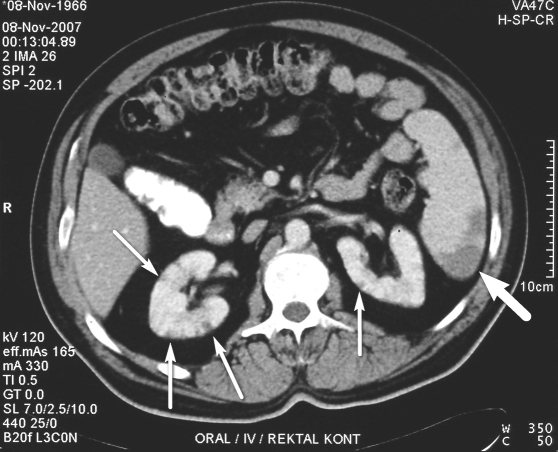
Abdominal CT shows multiple infarcts in kidneys (thin arrows) and spleen (thick arrow) just after the bronchial artery embolization.
